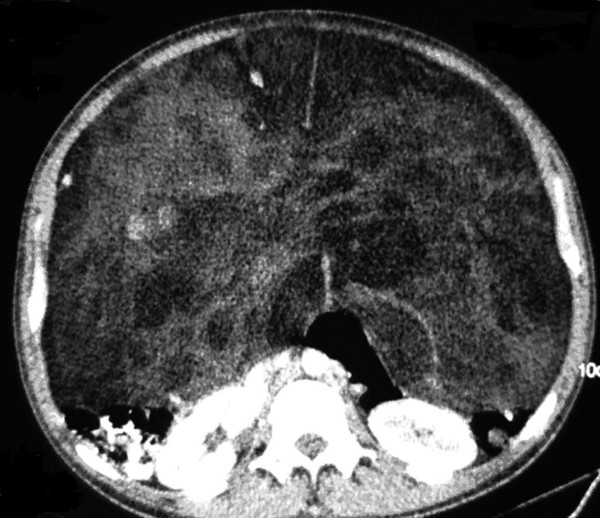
CT scan. Heterogeneous lesion is observed with zones of fat and solid density that entirely occupy the abdominal cavity, displacing retroperitoneal structures dorsally.
